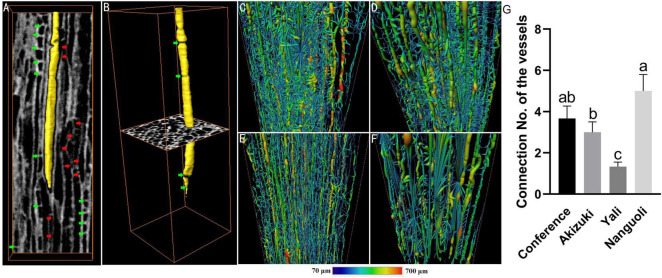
Structural and spatial arrangement of xylem vessels in the pedicel by X-ray scanning. (A) Longitudinal slice of the vessel tissue, light gray areas indicate the vessel walls, dark gray areas indicate the vessel lumens, one of which was rendered in yellow; red arrows indicate the location of intervessel connection; green arrows indicate the interruptions of the vessel lumen; (B) a representative 3D-reconstructed vessel isolated from surrounding vessels; panels (C–F) are the 3D-reconstructed skeleton of the vessels of Conference, Akizuki, Yali, and Nanguoli, respectively; (G) intervessel connectivity analysis, connection no. means the average no. of intervessel connections. Bars with different letters on the top are significantly different at *p* < 0.05 using the Tukey’s multiple range test.
